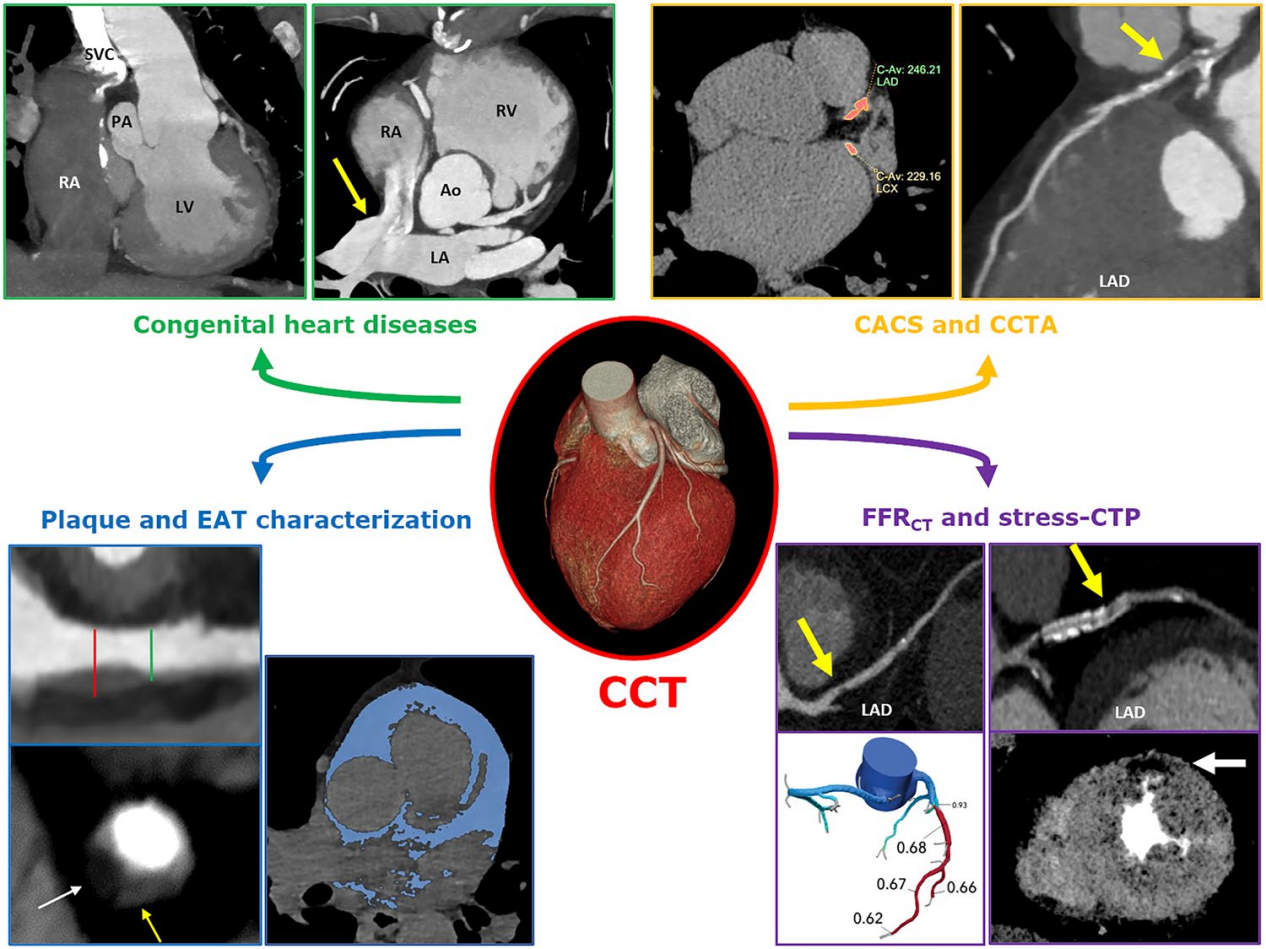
Graphical overview of the main applications of Cardiac Computed Tomography discussed in this part I appropriateness criteria guidelines from SIRM-SIC
